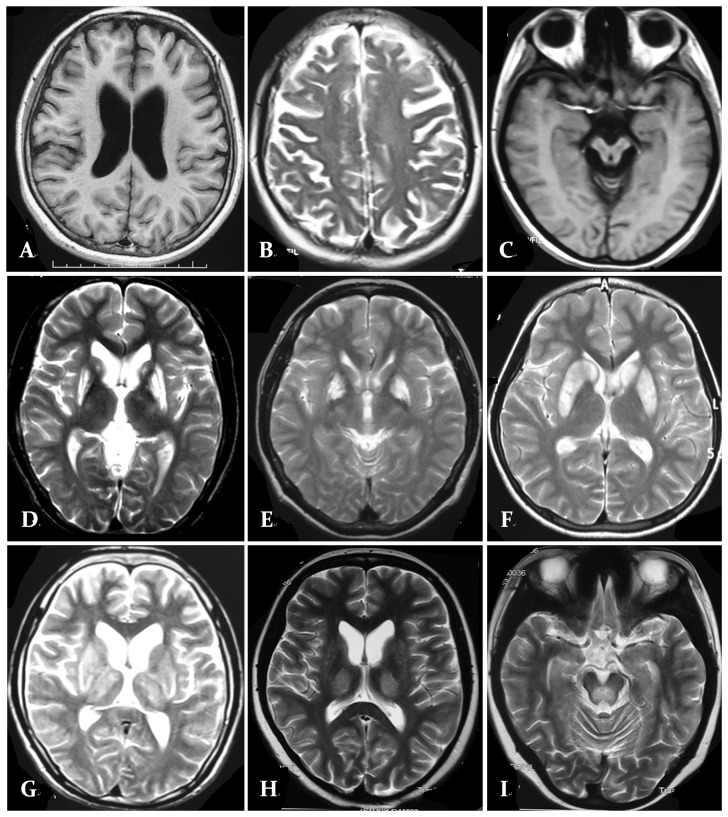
MRI with classically described changes in patients with Wilson 's disease.
